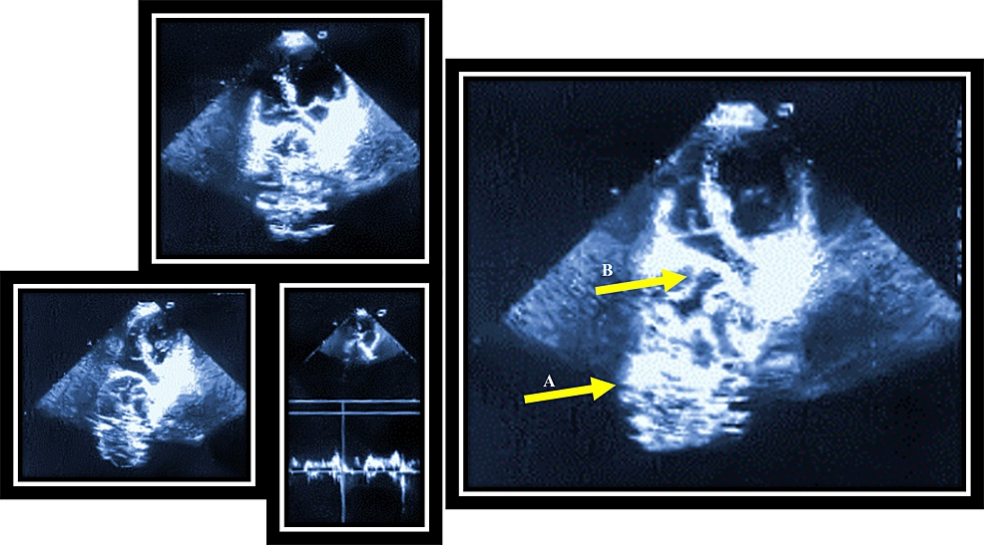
TTE 4-chamber views showing huge extracardiac mass compressing RA and RV, with altered diastolic filling. Arrow A,- extracardiac mass; arrow B, right atrium compression; TTE, transthoracic echocardiography; RA, right atrium; RV, right ventricle
